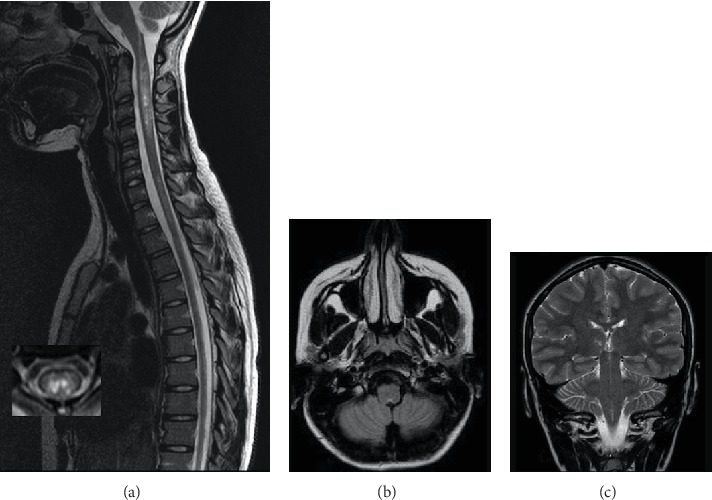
(a) Sagittal and axial T2-weighted spinal MR imaging showing hyperintense lesions longitudinally extended from C1 to C5 and from C6 to T6, involving the central spinal cord, with bright spotty lesions. (b) Axial T2-weighted fluid-attenuated inversion recovery (FLAIR) with hyperintense lesion in the brainstem involving the dorsal medulla (area postrema). (c) Coronal T1-weighted brain MRI shows a lesion on the right ventrothalamic area.
